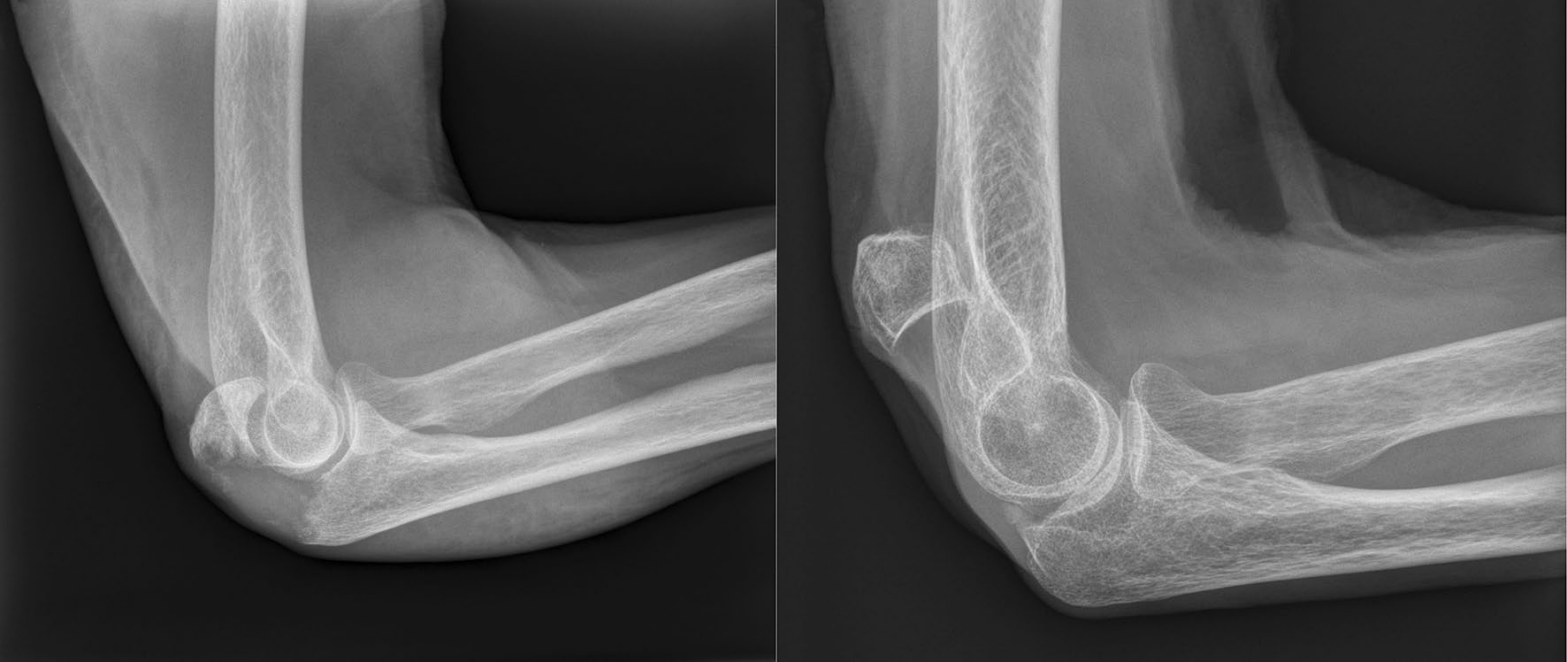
Lateral radiographs of a patient´s olecranon fracture (Mayo type IIA) on the injury day and at follow-up
